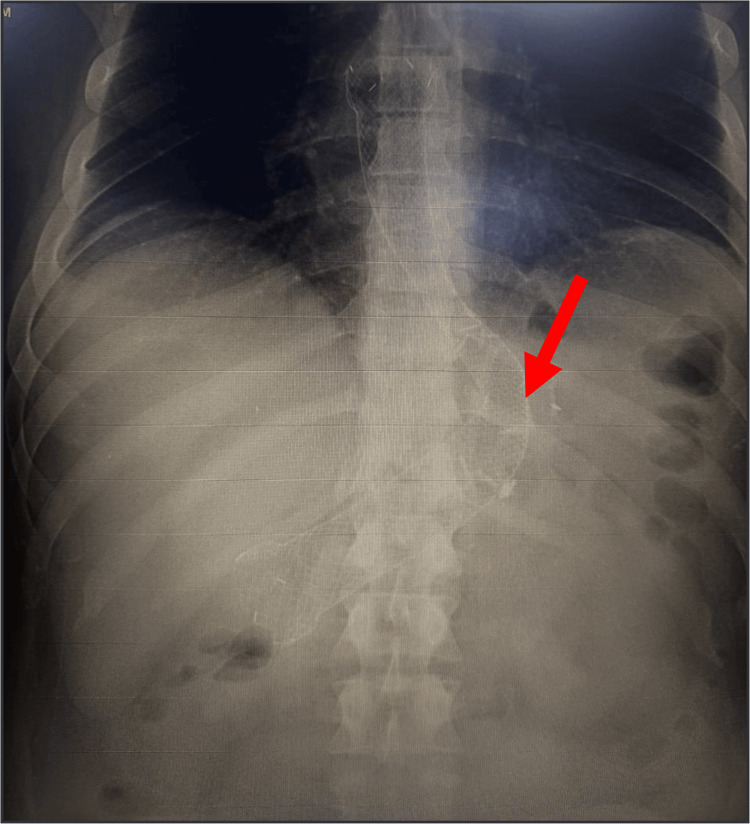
X-ray post stent insertion Plain abdominal radiograph following endoscopic mega-stent insertion (Day 19) confirming stent positioning across the staple-line defect.
